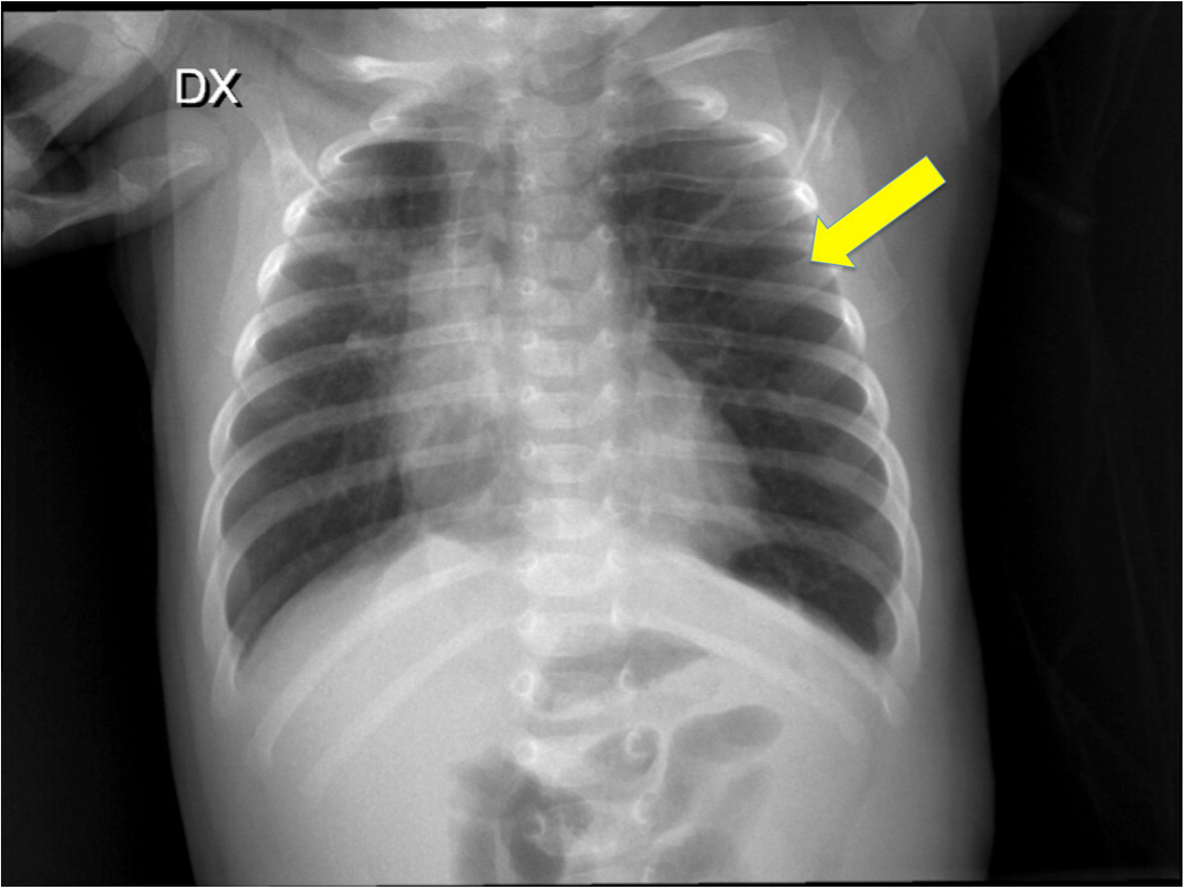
Chest X-ray at admission shows left pulmonary areas of hyperinflation (see arrows)
